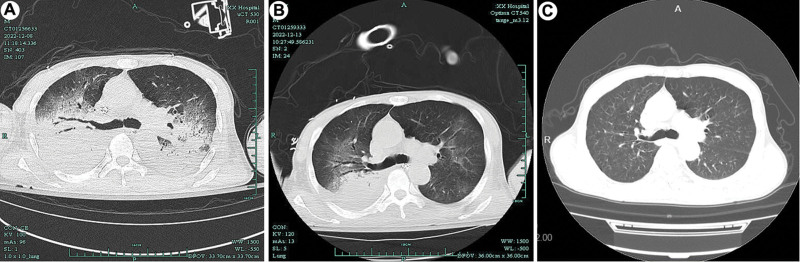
Chest CT scan on December 8, 2022 (A), December 12, 2022 (B) and January 10, 2023 (C).
